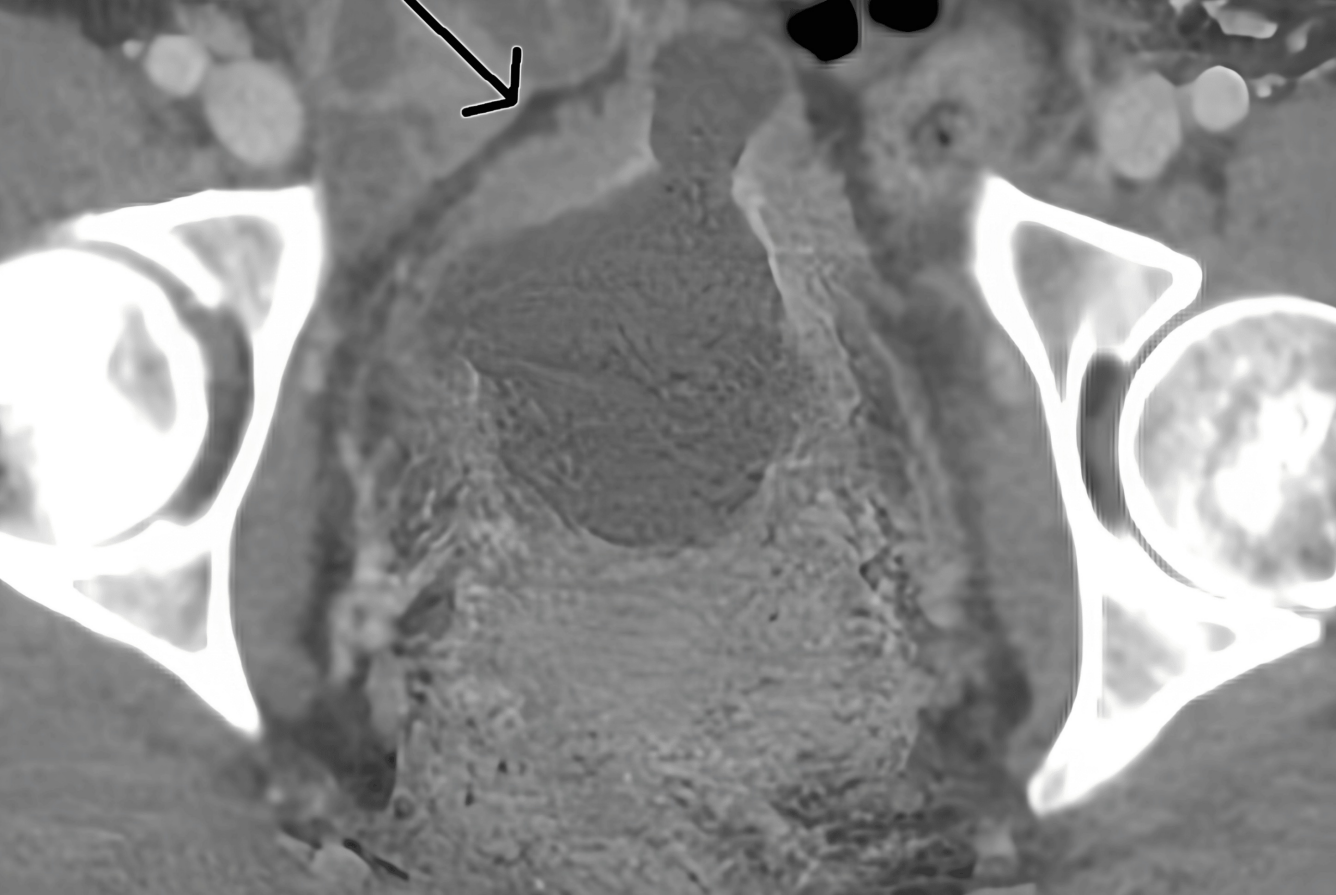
Axial CT scan of the pelvis demonstrating circumferential bladder wall thickening (arrow), a radiological feature suggestive of chronic inflammatory changes associated with ketamine-induced hemorrhagic cystitis
